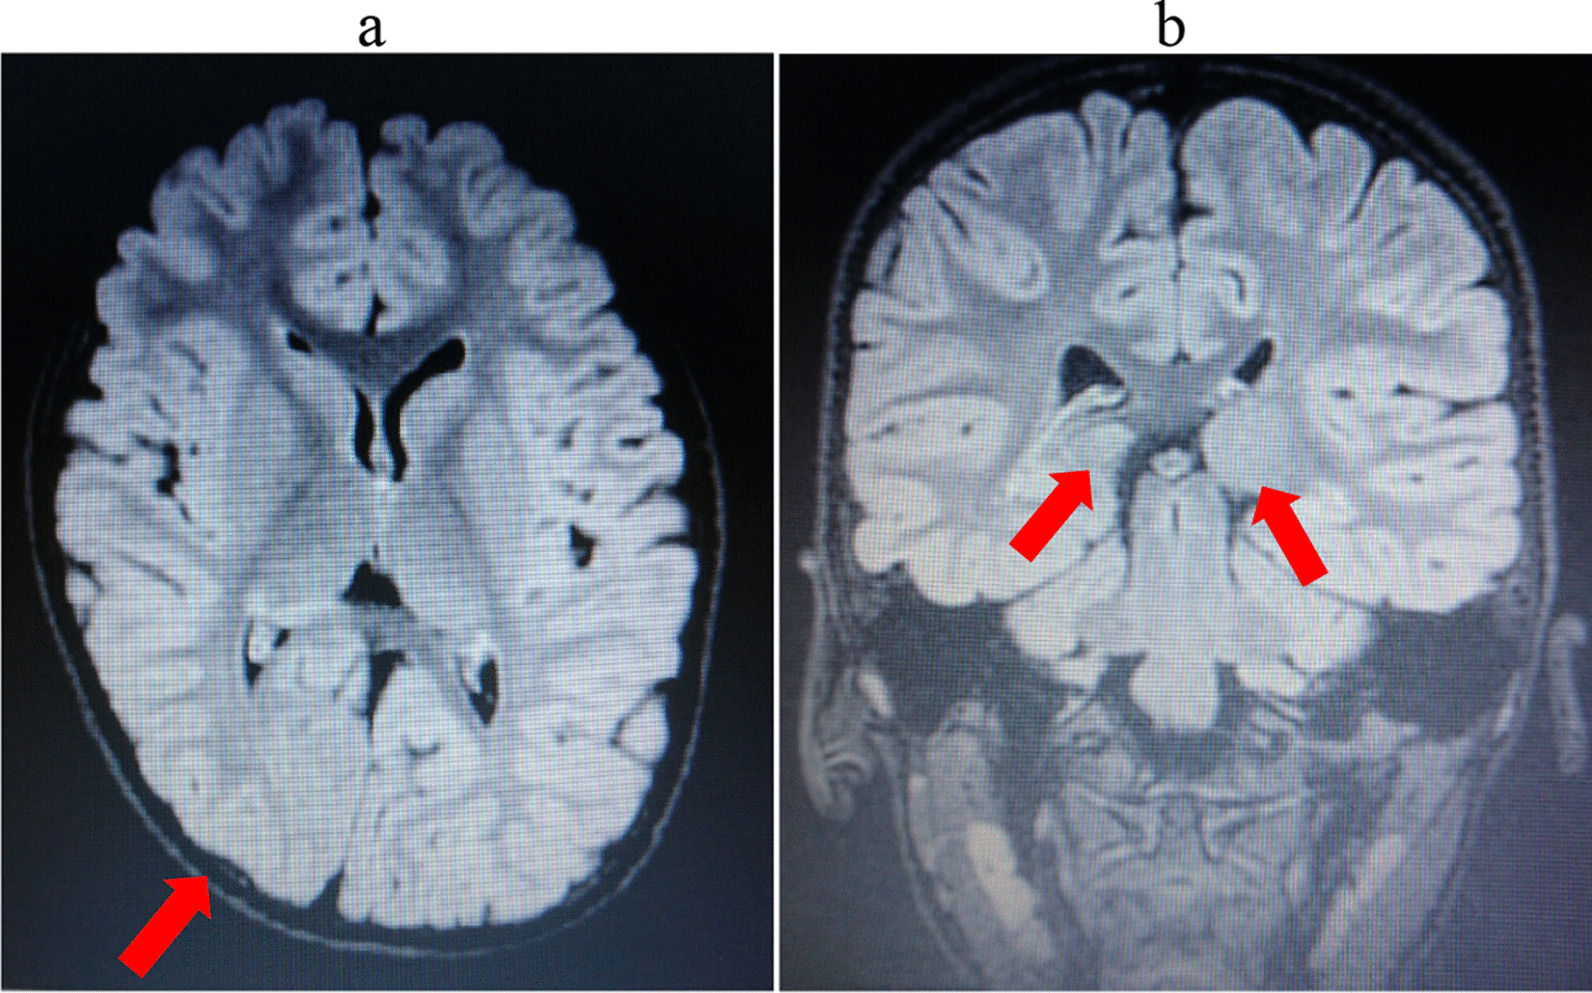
A T2-weighted magnetic resonance image of the brain showed (a) laterality of the right occipital lobe (red arrow) and (b) volume reduction of the right thalamus (red arrows show thalami)
